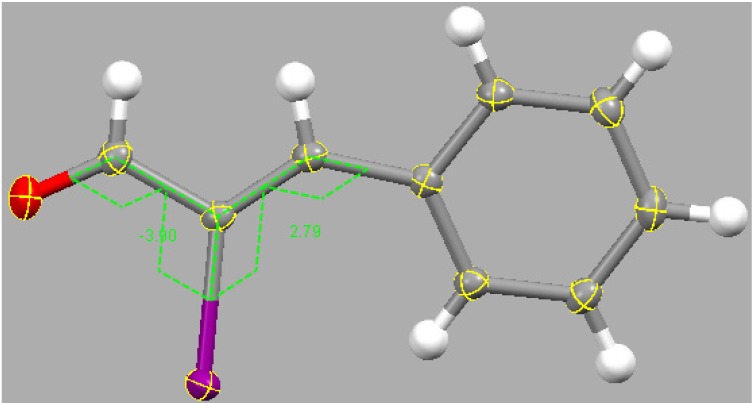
X-ray molecular structure of the title compound including the two torsion angles, −3.9° and 2.8° surrounding the iodine. These values, although small, are greater than the unsubstituted cinnamaldehyde [26] (1.06° and 0.85°, respectively) due to the bulky iodine atom.
